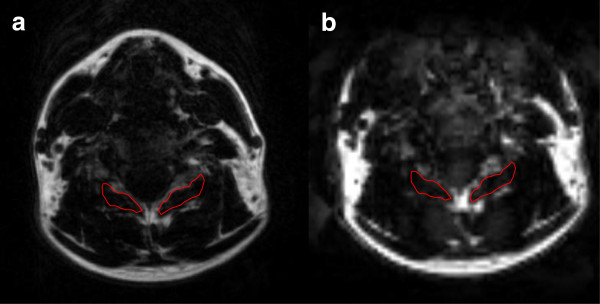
Regions of interest measures of right and left multifidus muscle at the C5 segmental level; a) T1-weighted magnetic resonance image b) fat-only image derived from the 3D VARPRO acquisition. Images were co-registered.
